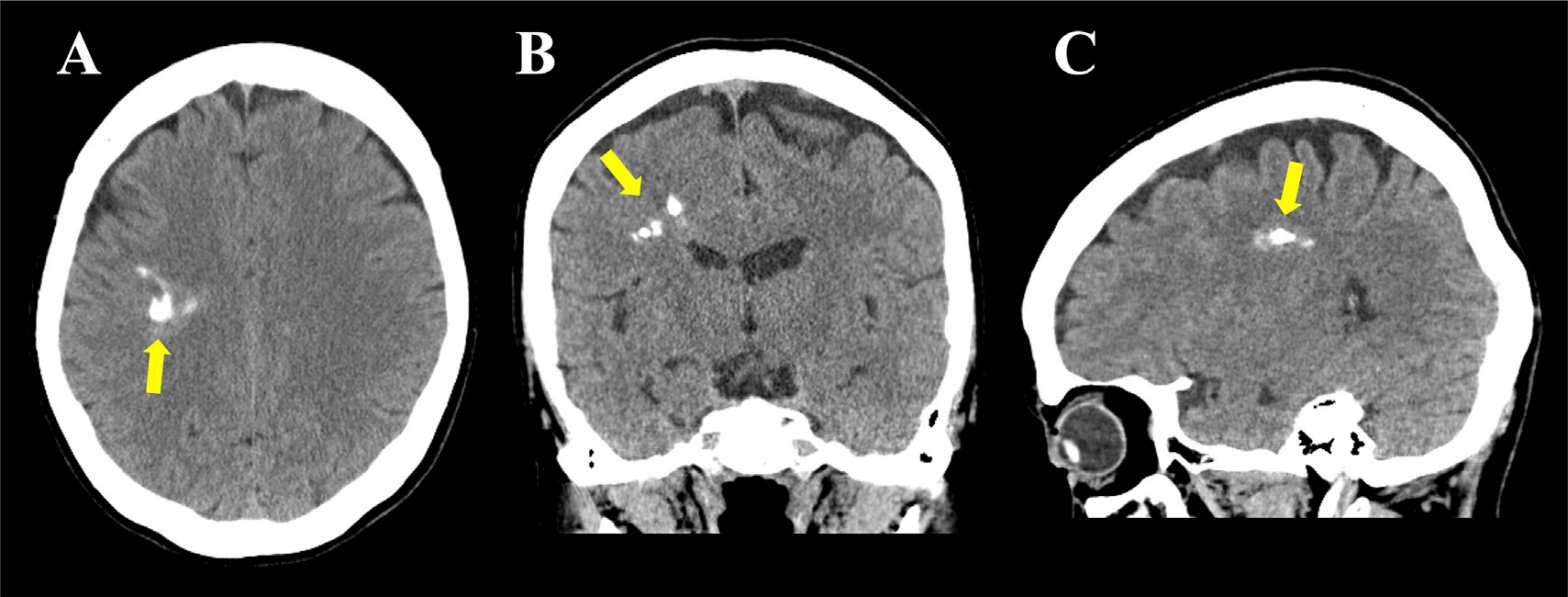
Head CT findings. Noncontrast CT images of the head in the axial (A), coronal (B), and sagittal (C) views show irregular and heterogeneous coarse calcifications (arrows) in the deep white matter of the right frontal and parietal lobes.
